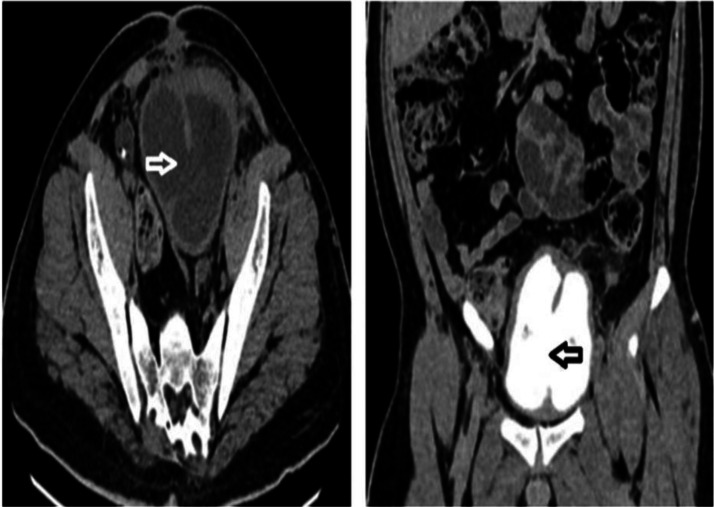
Follow-up CT urogram images: axial non contrast phase image demonstrating the post-operative resected septum, establishing communication between the bladder compartments (annotated by white arrow). Coronal delayed phase image demonstrating the post-operative resected septum (annotated by black arrow).
